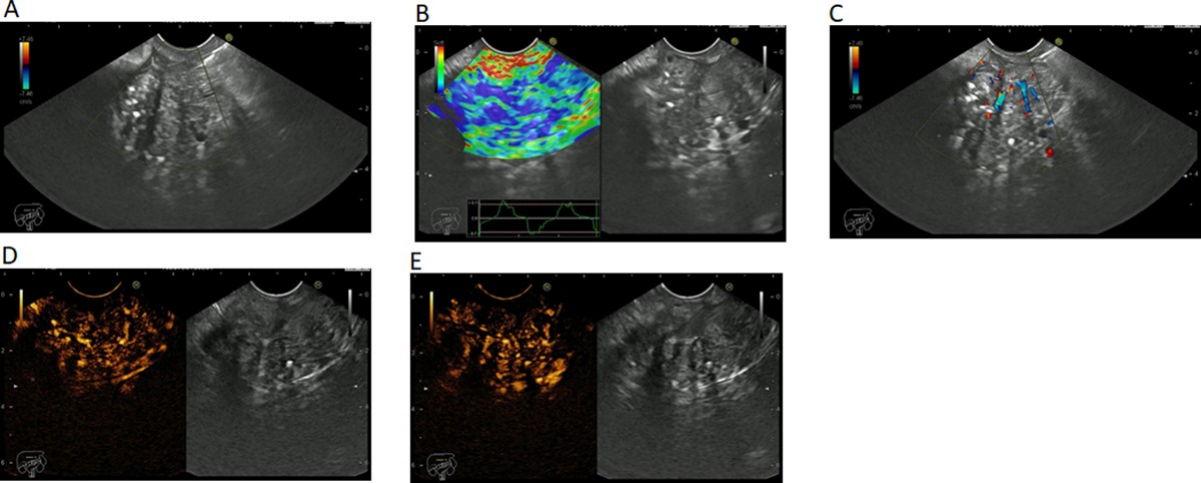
EUS imaging of a pseudotumoral chronic pancreatitis in (A) gray scale. (B) elastography. (C) color Doppler. (D) contrast enhancement–arterial phase. (E) contrast enhancement–venous phase.
